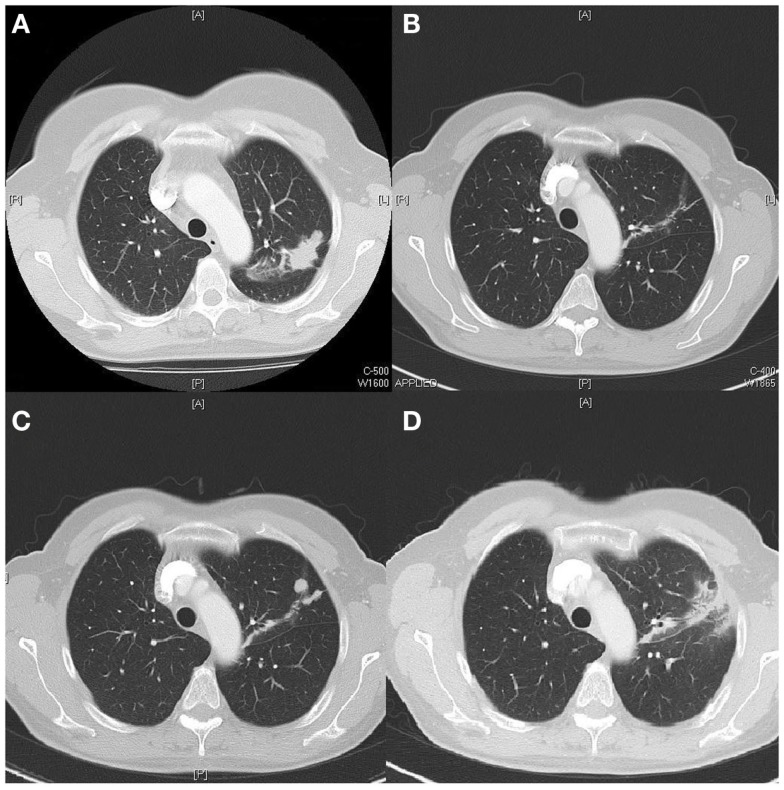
(A) Tumor upper left lobe on diagnosis (CT scan). (B) Near complete response 2 months after start TKI (CT scan). (C) Local progression 18 months after diagnosis (CT scan). (D) Partial response 3 months after local stereotactic radiotherapy (CT scan).
